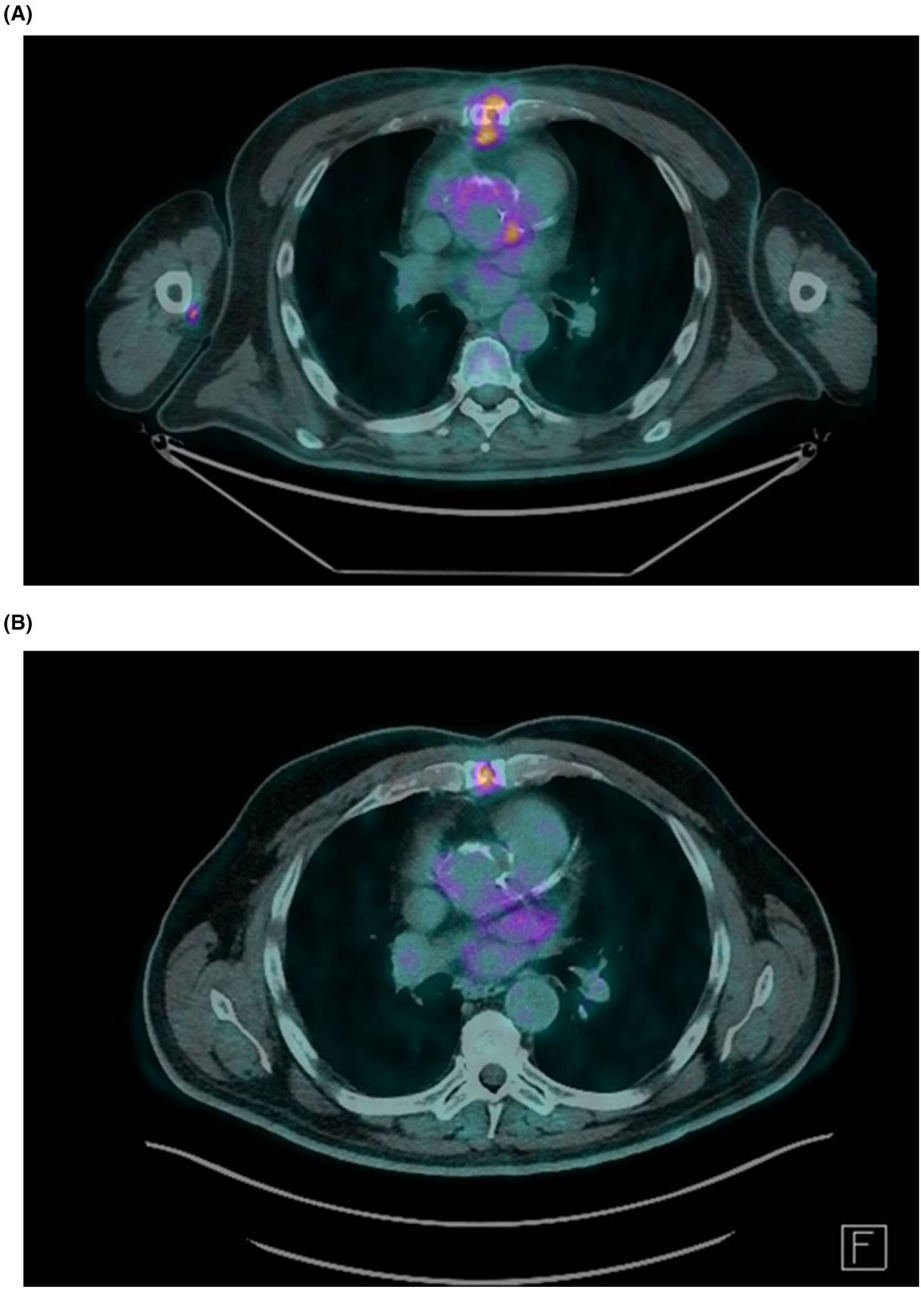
(A) Patient 9, initial PET‐CT scan showing increased tracer uptake (standardised uptake value, SUVmax 6) around the aortic root and in the anterior mediastinal fat (SUVmax 5), with hypermetabolic lymph nodes in the paratracheal and precarinal regions (SUVmax 5). Clinically, a sternal wound infection, mediastinitis and possible prosthetic aortic endocarditis with fluconazole‐resistant
